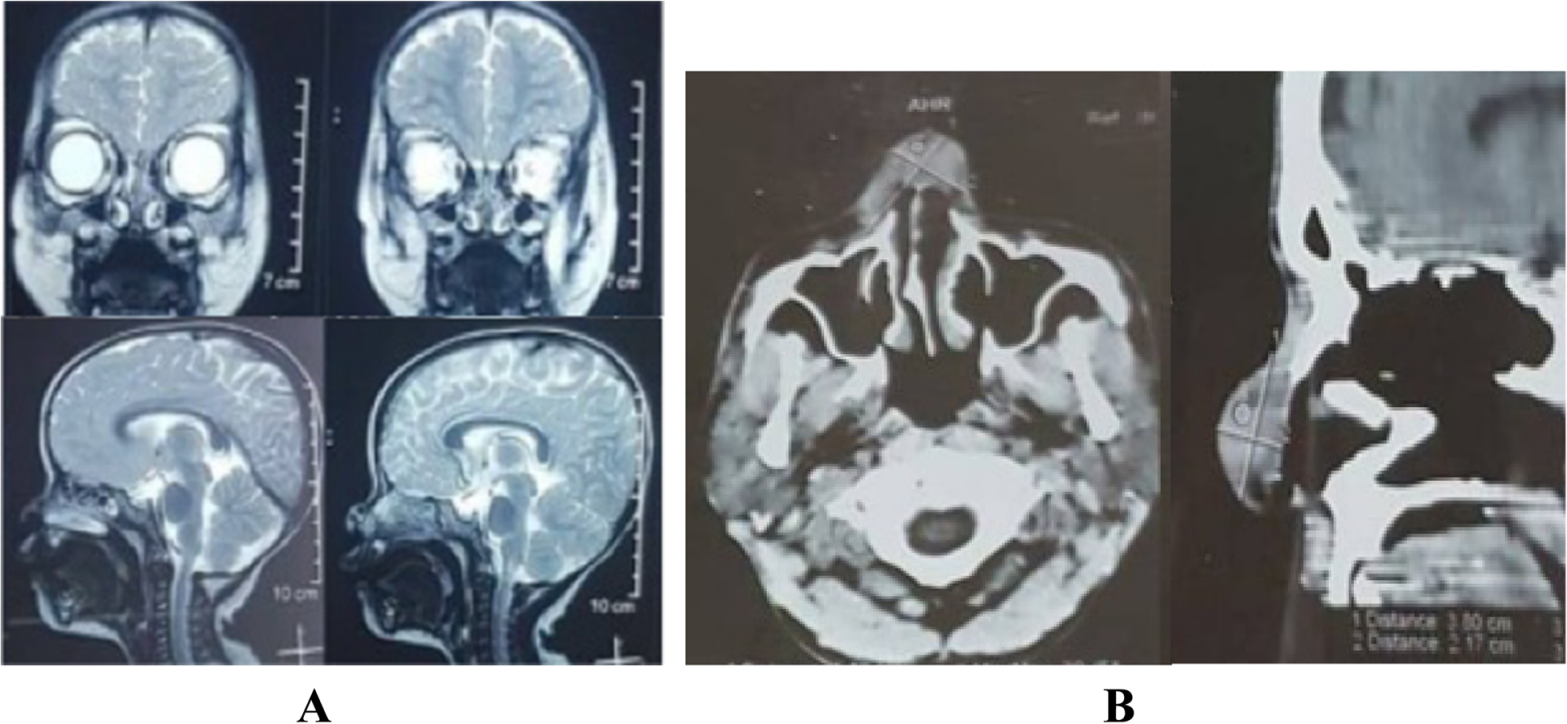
Computed tomographic scans of the patients. a Patient 1. b Patient 2
